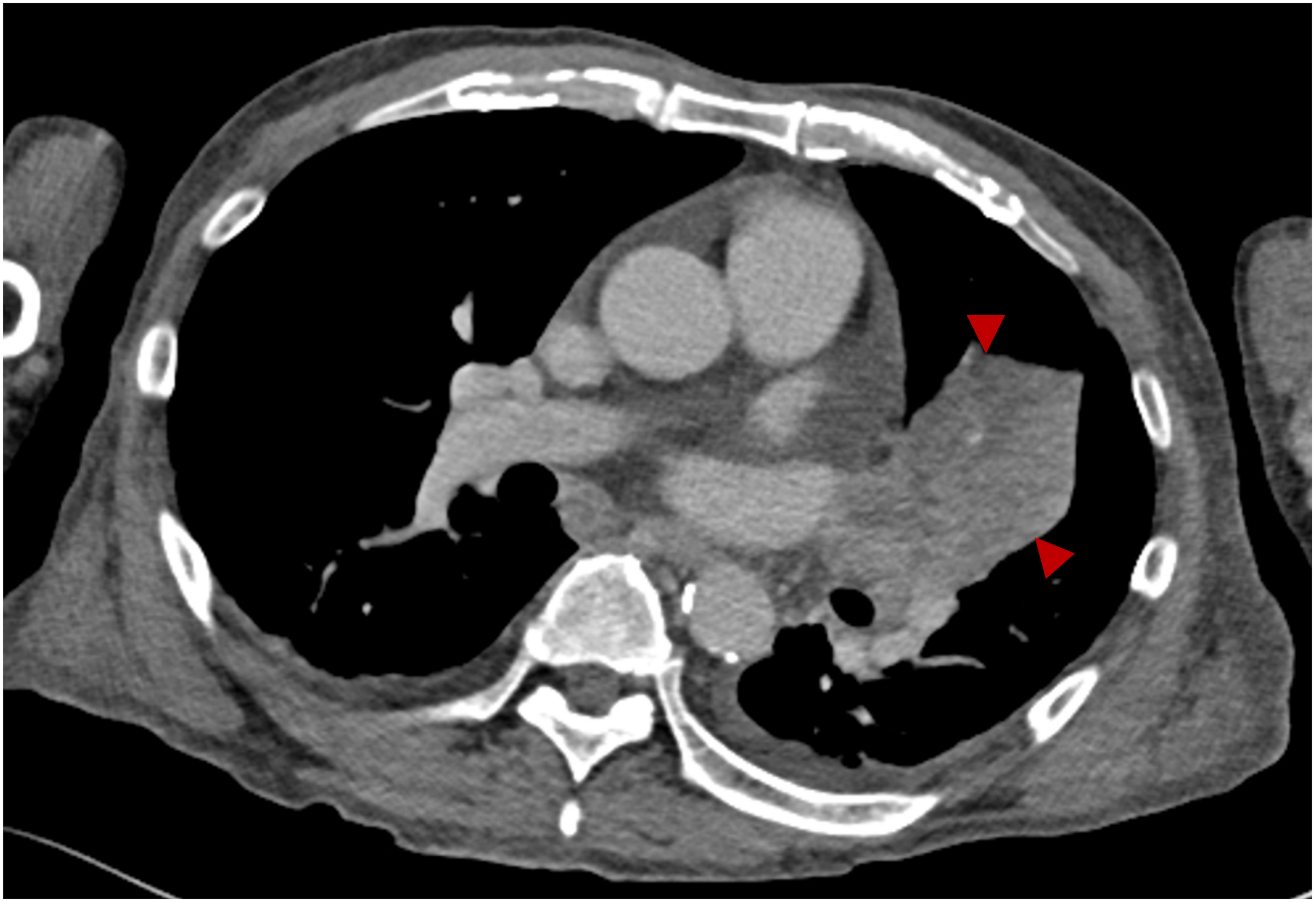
Contrast-enhanced computed tomography on admission. Contrast-enhanced computed tomography showing left upper lobe lung cancer (arrowheads).
